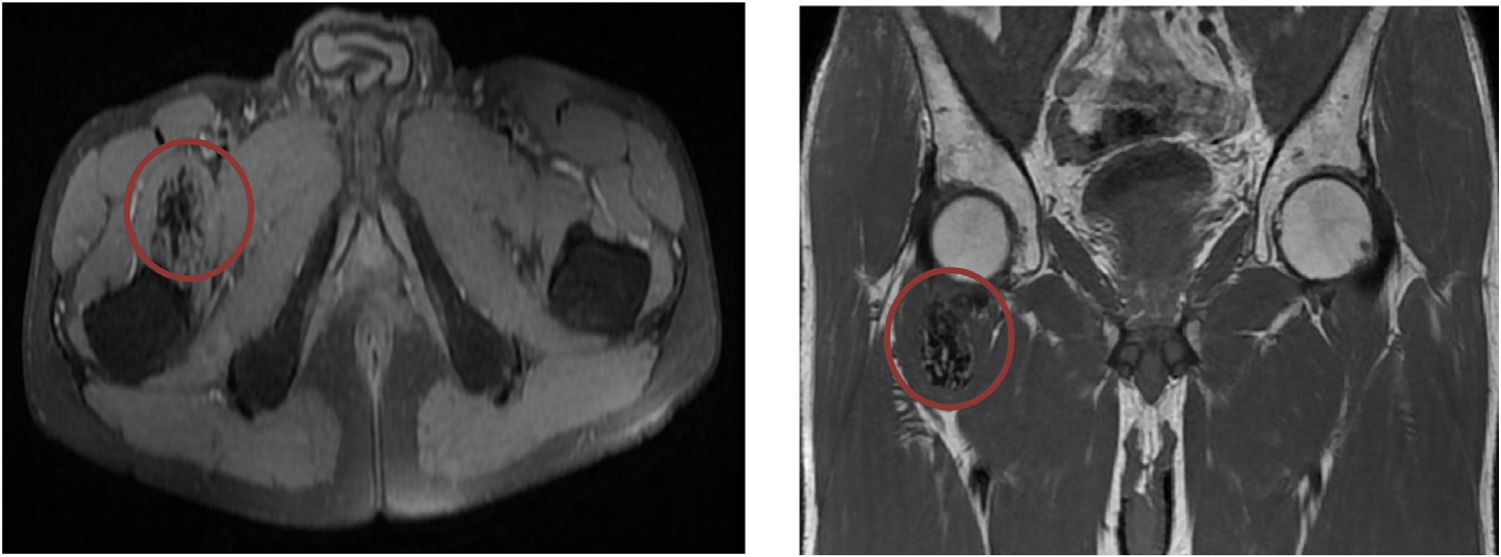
MRI of the pelvis on T1-weighted axial and coronal MRI, showing a lobulated mass of the right hip, well-delimited, and infiltrating the muscles, in hyposignal T1 (red circle).
